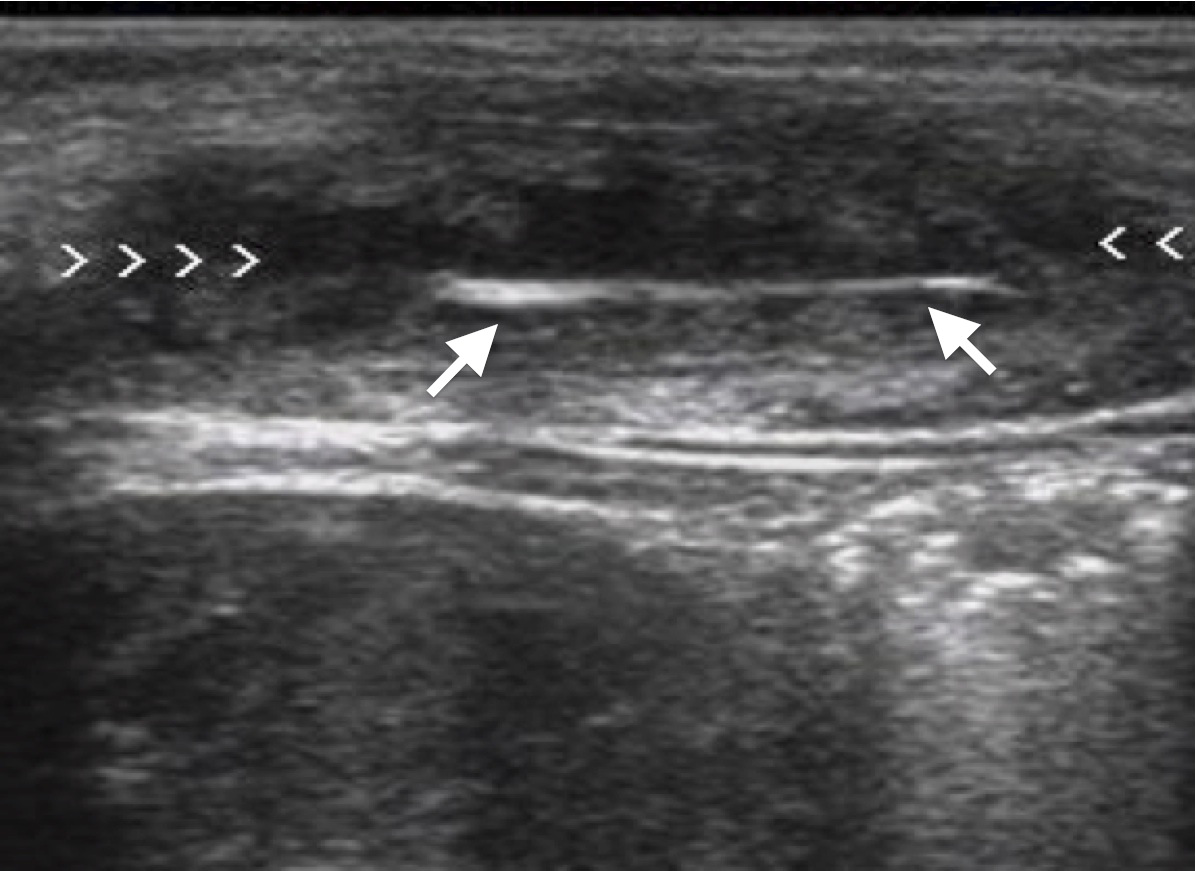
Longitudinal sonogram shows a hyperechoic 6 mm wooden foreign body (long arrow) without posterior acoustic but with surrounding halo sign (arrowhead) due to soft tissue abscess in the forearm.
